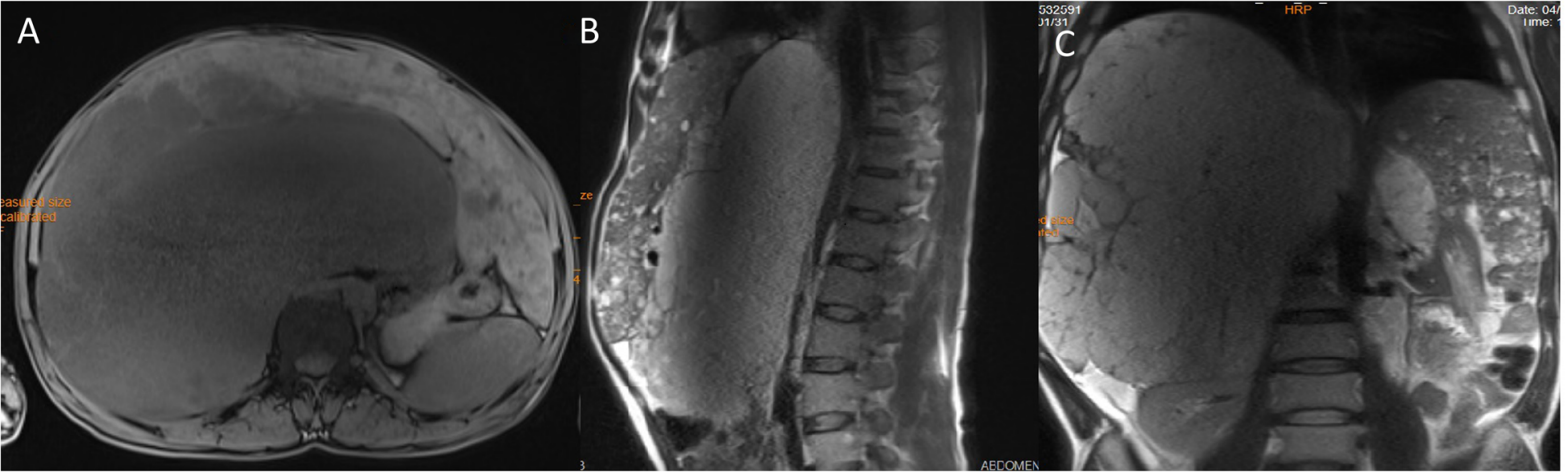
T1-weighted abdominal MRI showing a huge heterogeneous mass originating from right liver lobe in axial, sagittal and coronal views (a, b and c, respectively) with nodular discontinuous enhancement after gadolinium injection (b, c)
